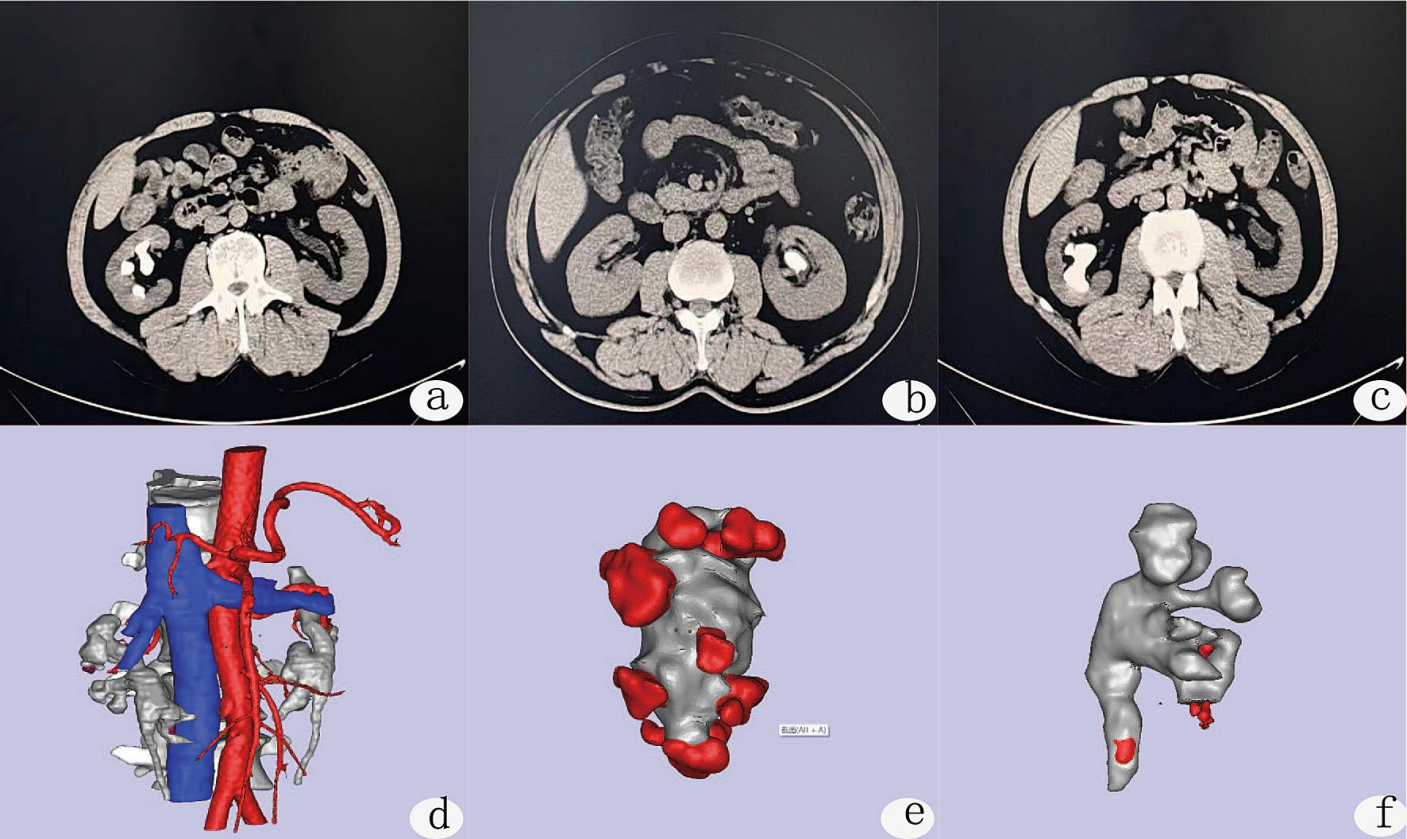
CT and three-dimensional reconstruction of different parts of the kidney. (a–c) Typical preoperative CT of the patients; (d–f) three-dimensional reconstruction images of different parts of the kidney. (d) Distribution of renal blood vessels, (e) renal pelvis and calculi, (f) three-dimensional reconstruction of the renal pelvis and the size and location of the calculus.
